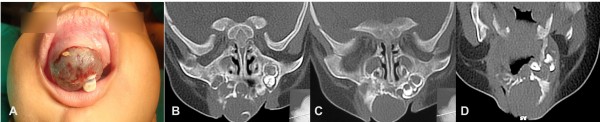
Prematurely erupted teeth with a firm, non-ulcerated, reddish-bluish tumor approximately 4 cm × 4 cm in size, extending from the alveolar ridge to the hard palate and covered by intact mucosa. A, B, C, D: Pre-operative axial computed tomography scans showing an erosive and expansive lesion extending from the alveolar ridge to the hard palate and regional displacement of teeth.
